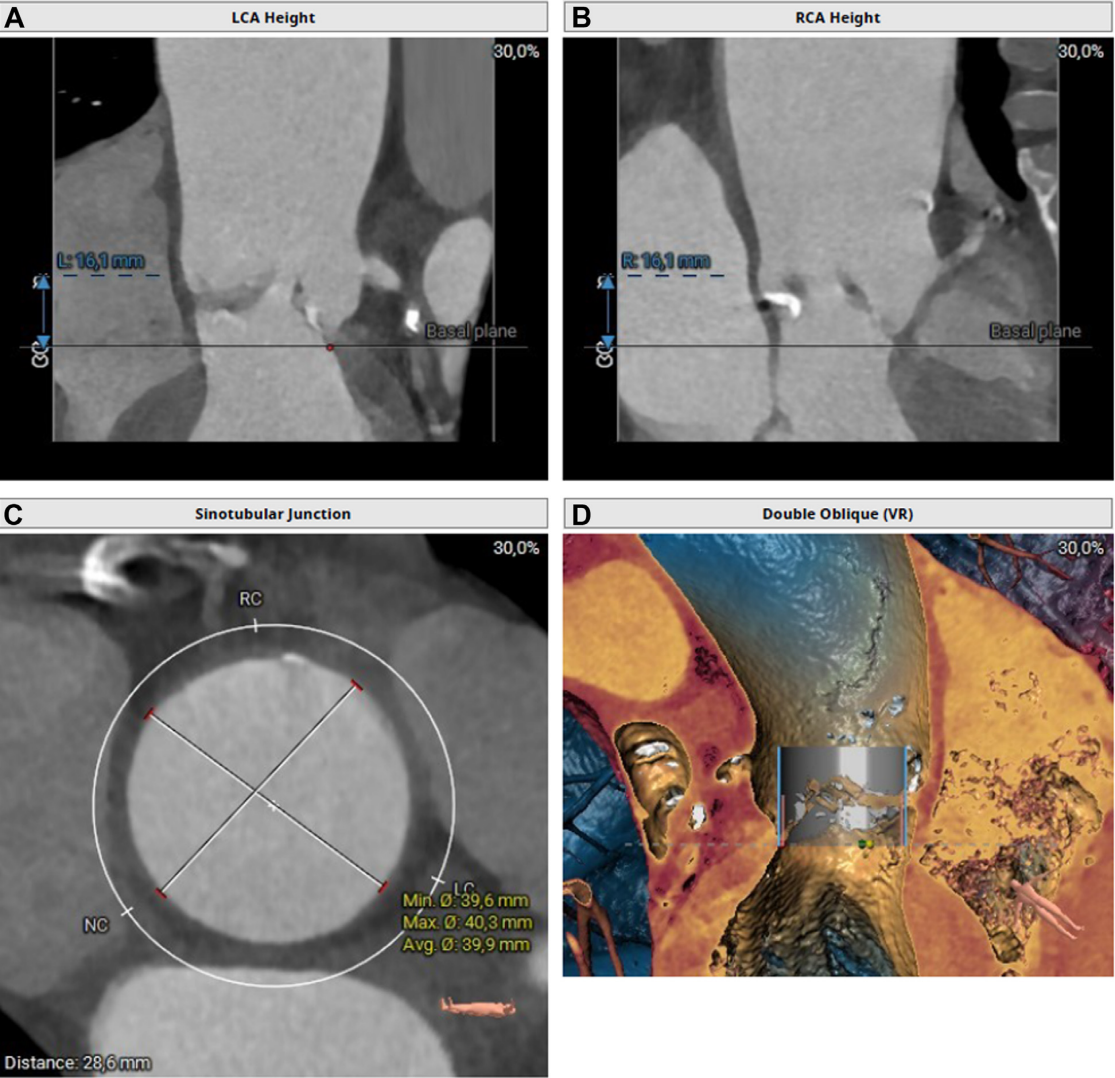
Coronary Heights and Sinotubular Junction Measurements on Preoperative CT (A and B) LCA and RCA heights from the basal plane. (C) Virtual rendering with an Edwards Sapien 3 bioprosthesis in the proper position. (D) Sinotubular junction measurements. CT = computed tomography; LC = left coronary cusp; LCA = left coronary artery; NC = noncoronary cusp; RC = right coronary cusp; RCA = right coronary artery.
